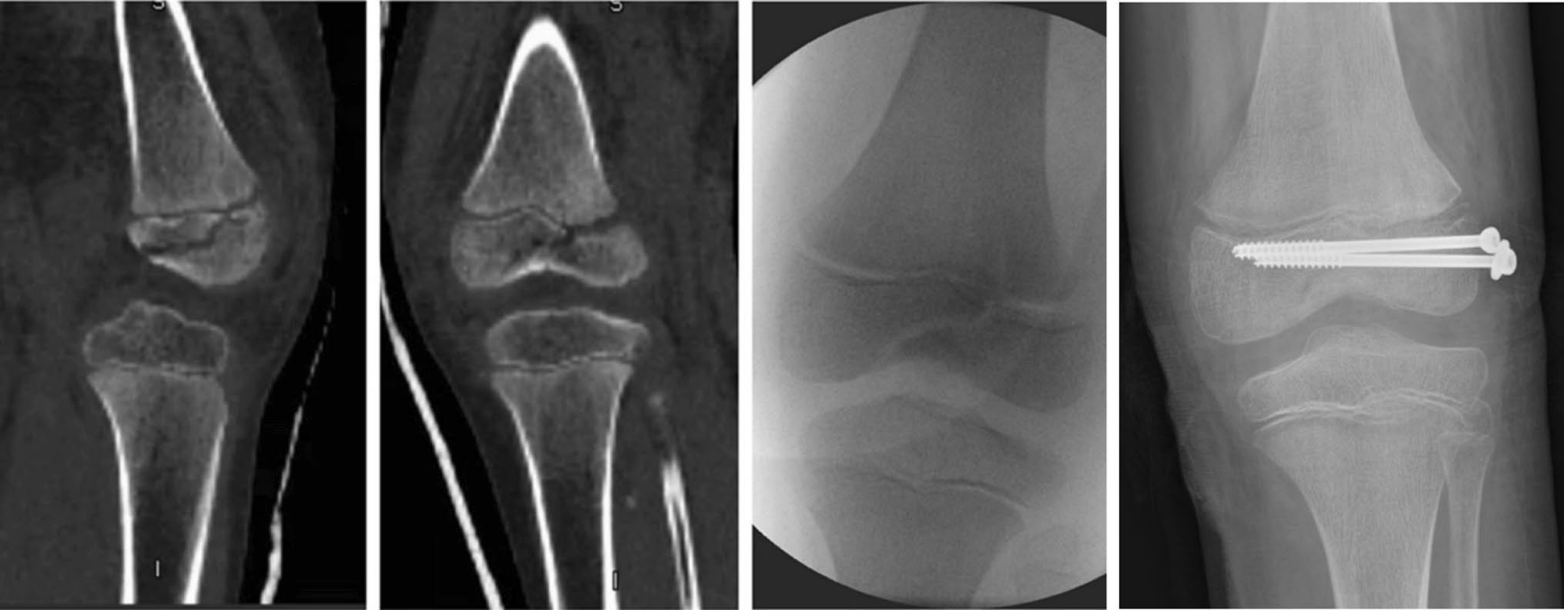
CT scan and intraoperative image demonstrating a SH III fracture with subsequent screw fixation
